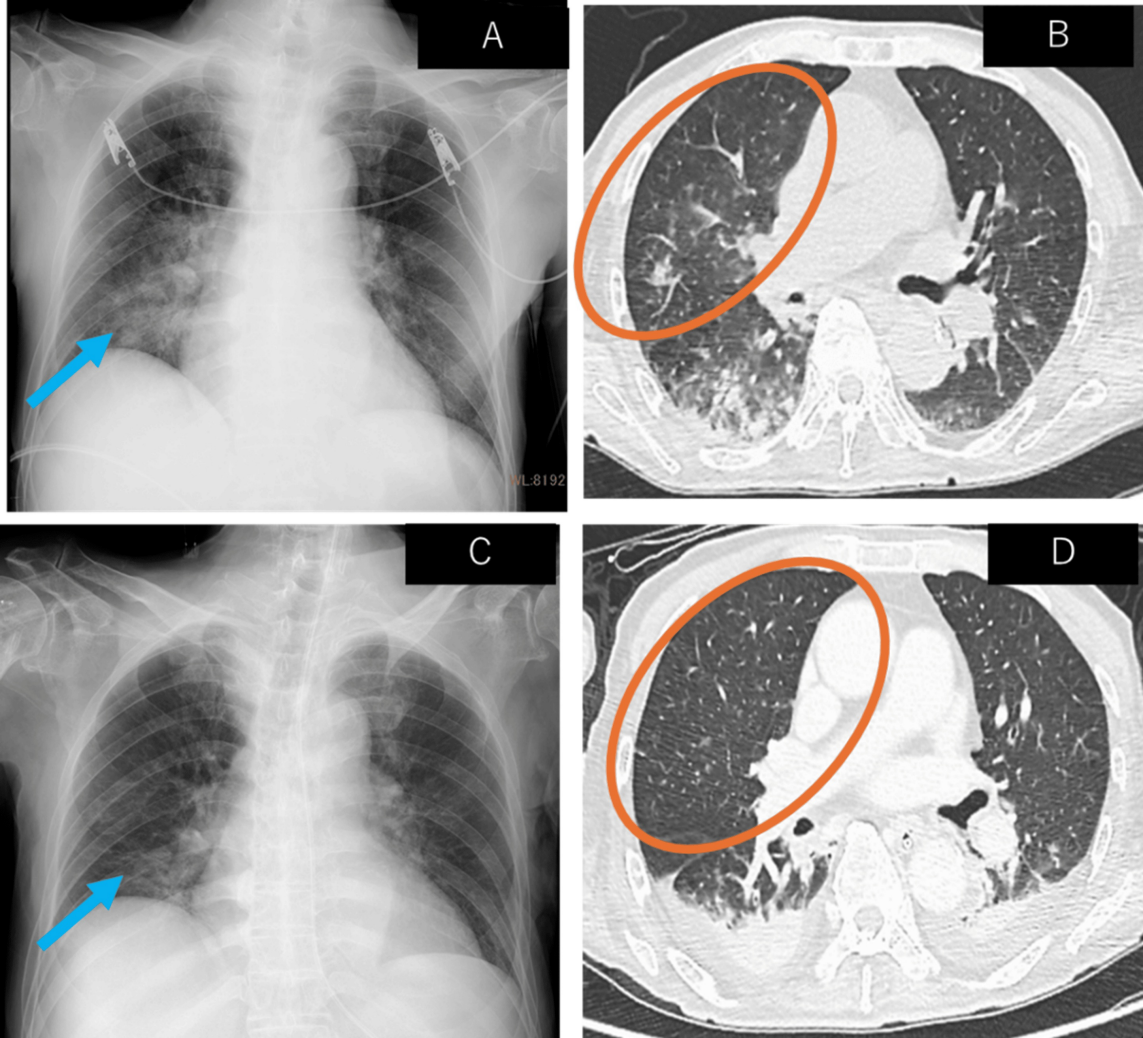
Chest radiography and computed tomography images (A) Radiography showed ground-glass opacities in the right lower lung field on day 1. (B) Computed tomography (CT) showed ground-glass opacities in the right upper and lower lobes on day 1. (C and D) The abnormal shadows observed on radiography and CT scans on day 1 were improved by day 6
